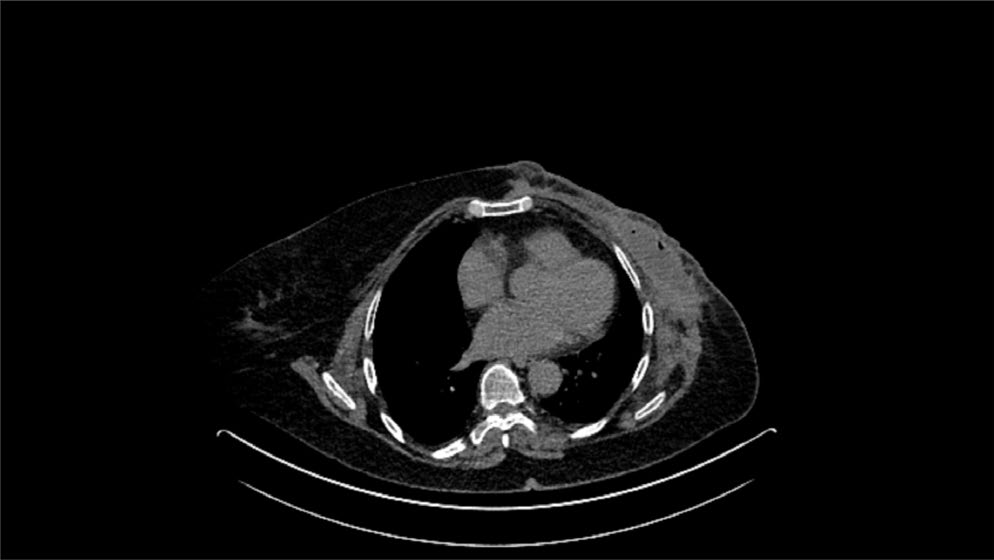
Thoracic CT scan in May 2022 reveals metastases in the left chest wall.
